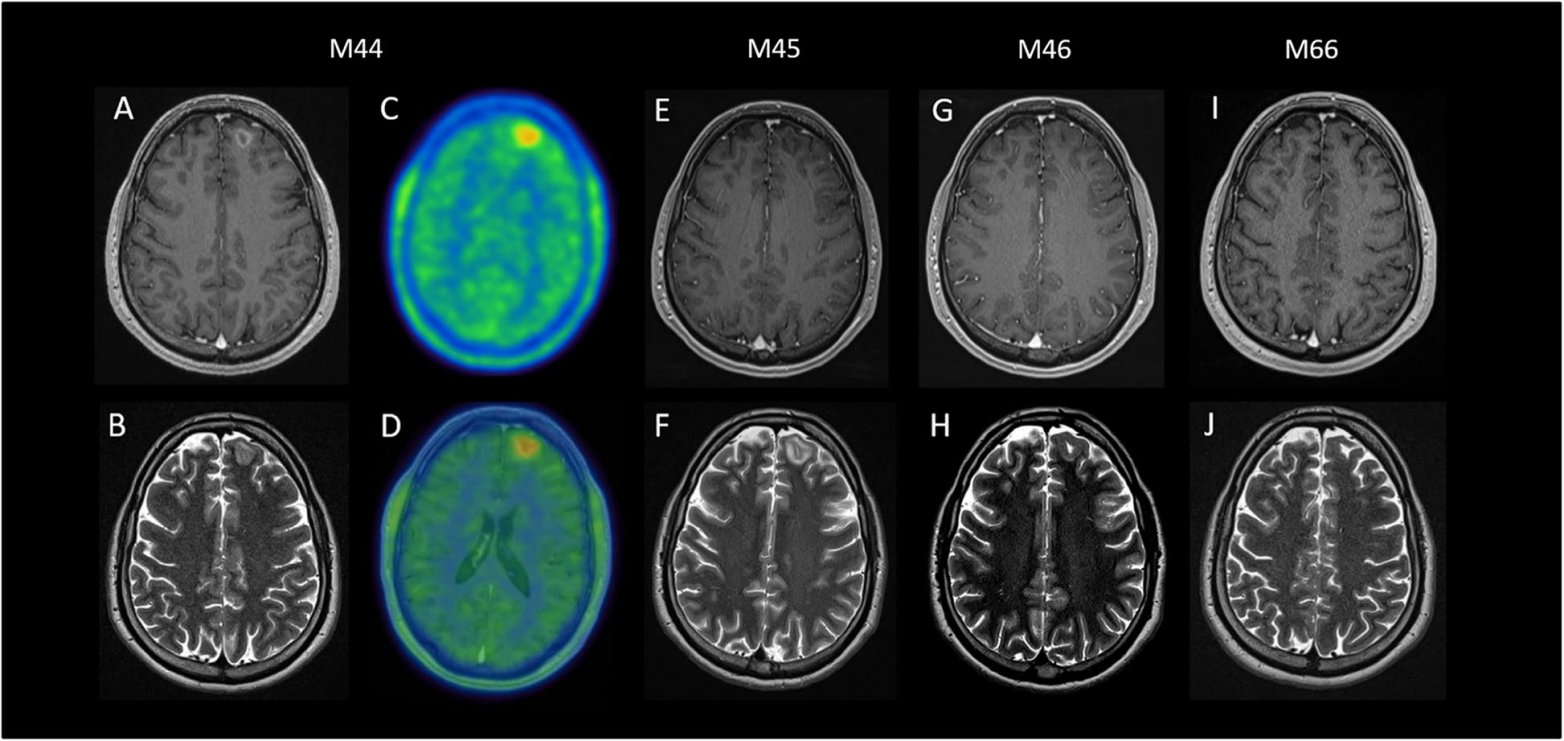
Excerpts of MRI and PET scans performed. Legend: The cerebral MRI scan during the demyelinating event at month 44 revealed a T1 hypointense, Gd enhancing (A), T2 hyperintense (B) lesion in the left Gyrus frontalis, which shows hypermetabolism in the FET-PET scan (C: original FET-PET image, D: MRI fused FET-PET image; SUV max: 2.64). MRI showed regression of lesions over time until month 66 (E, G, I: contrast-enhanced T1-weighted imaging. F, H, J: T2-weighted imaging). Abbreviations: FET-PET/CT = O-(2[18F]fluoroethyl)-L-tyrosine—positron emission tomography / computer tomography; Gd = Gadolinium; M = Month; MRI = Magnetic resonance imaging
